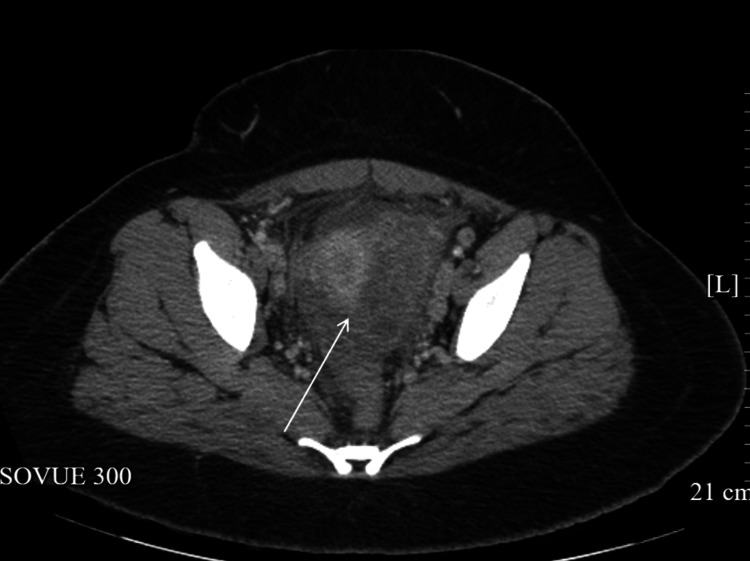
Follow-up CT of the abscess status post-drainage. The arrow points toward the resolving abscess.
